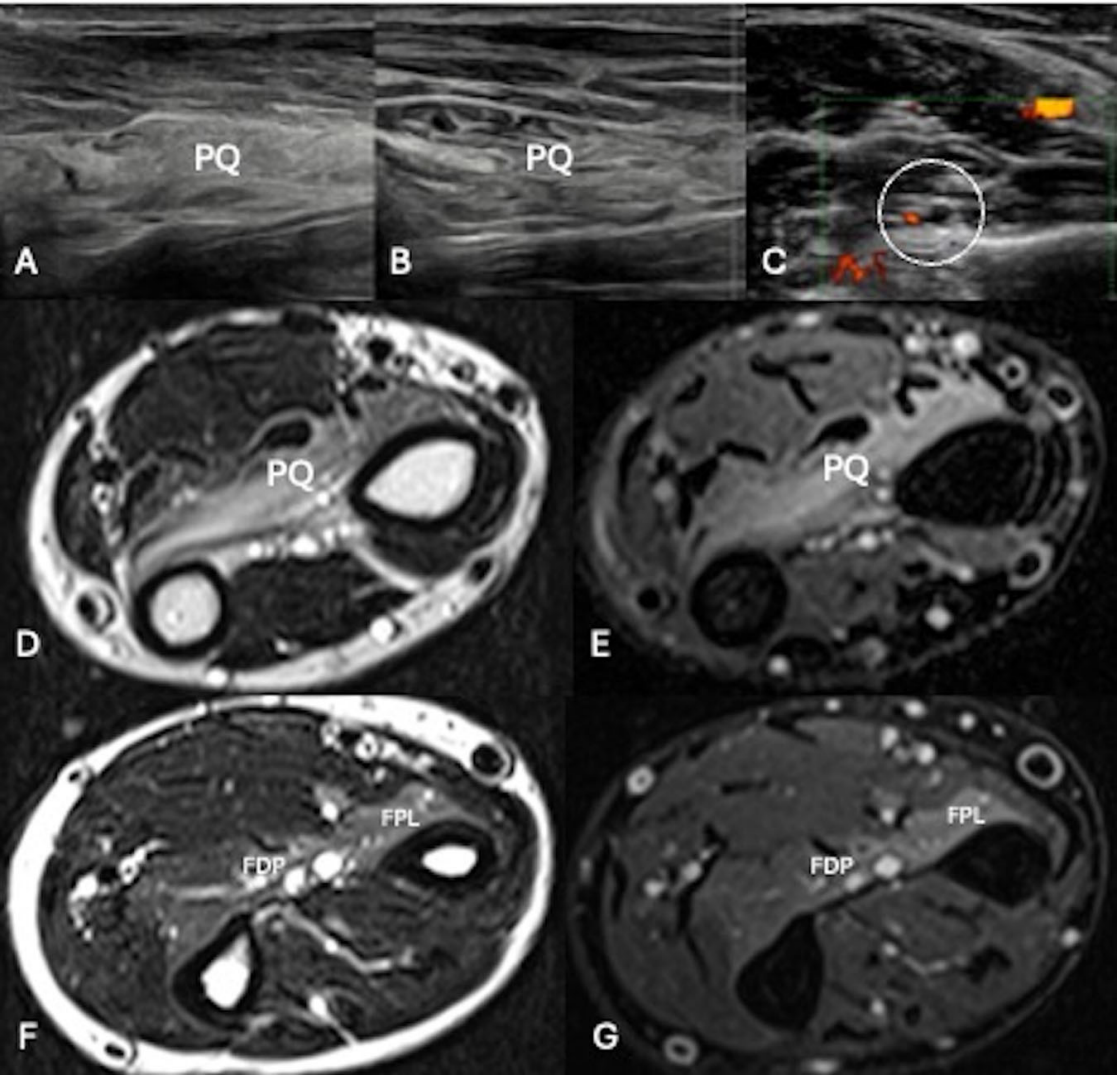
US and MRI images AIN syndrome. US images of denervation (A) of the pronator quadratus muscle, which appears thinner and diffusely hyperechoic, versus US normal appearance of the muscle in the unaffected side (B). Axial image of the volar aspect of the mid-third of the forearm (C) shows the thickening of the AIN running close to the anterior interosseous artery (circle). Axial T2-weighted (D, F) and axial fat-suppressed PD (E, G) MRI images show edema of pronator quadratus muscle (PQ), the radial half of the flexor digitorum profundus (FDP), and the flexor pollicis longus (FPL) due to denervation
